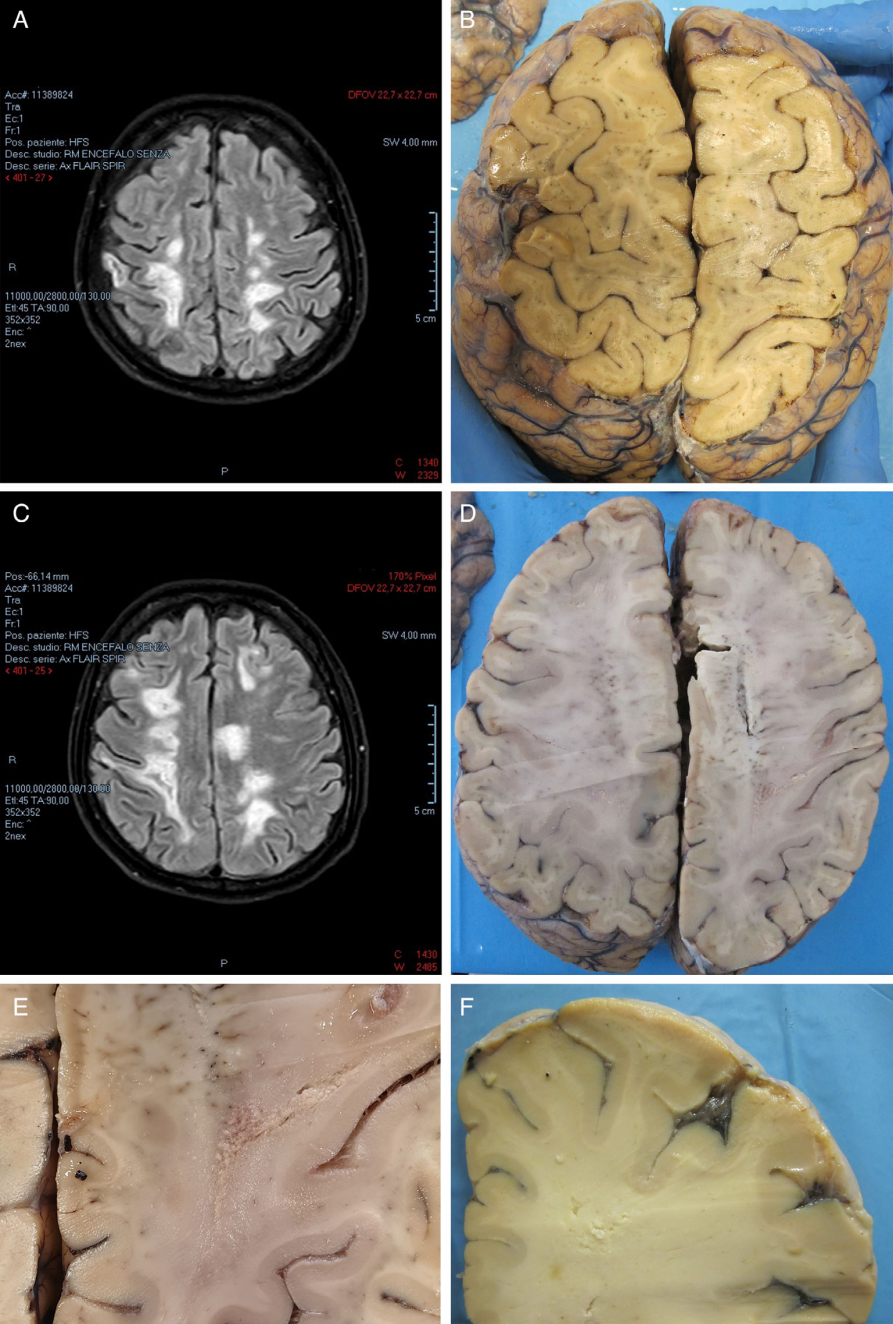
MRI (A, C) and gross (B, D‐F) findings of the cerebral hemispheres. (A, C) Axial FLAIR images taken on day 88 after first hospital admission show hyperintense areas in the cerebral white matter. (B, D) Horizontal brain slices corresponding to the imaging views (A, C) show the appearance of white matter lesions. (E, F) Details of lesions at higher magnifications are shown. Panels E and F correspond to selected area of slice D and taken at another slice.
